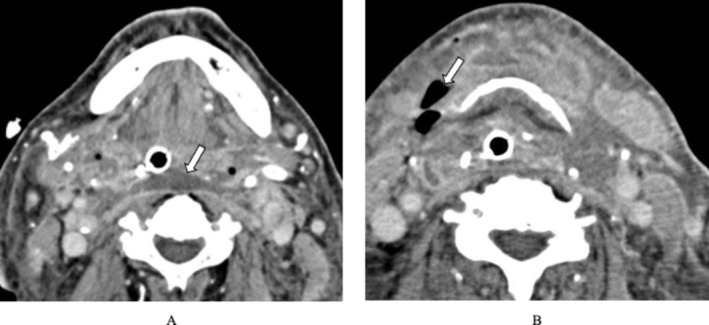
Contrast‐enhanced CT images on hospital Day 12. An abscess extending from the left submandibular gland to the anterior surface of the cervical vertebrae is observed (A, arrow), along with air‐containing lesions within the soft tissue (B, arrow).
